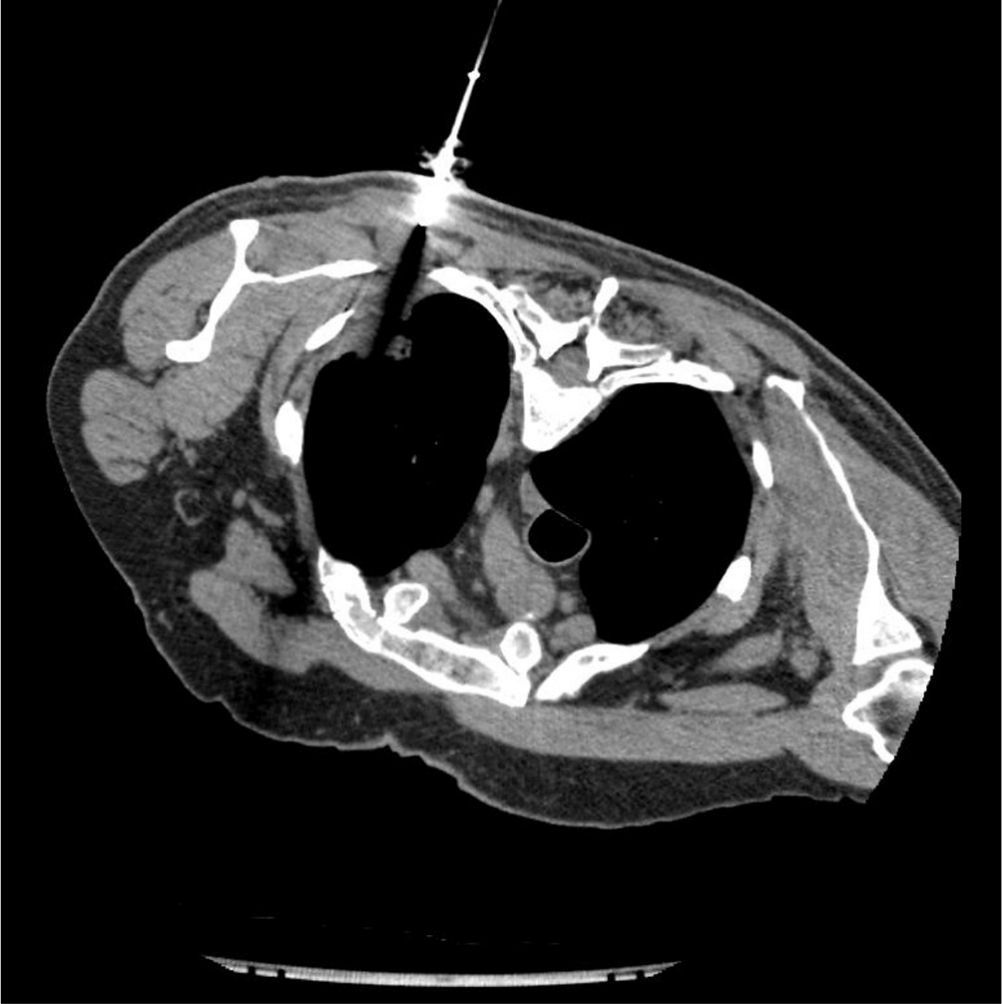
T*_1 _weighted sagittal MRI depicting the large mass infiltrating the thoracic vertebral bodies (T4/5/6).
